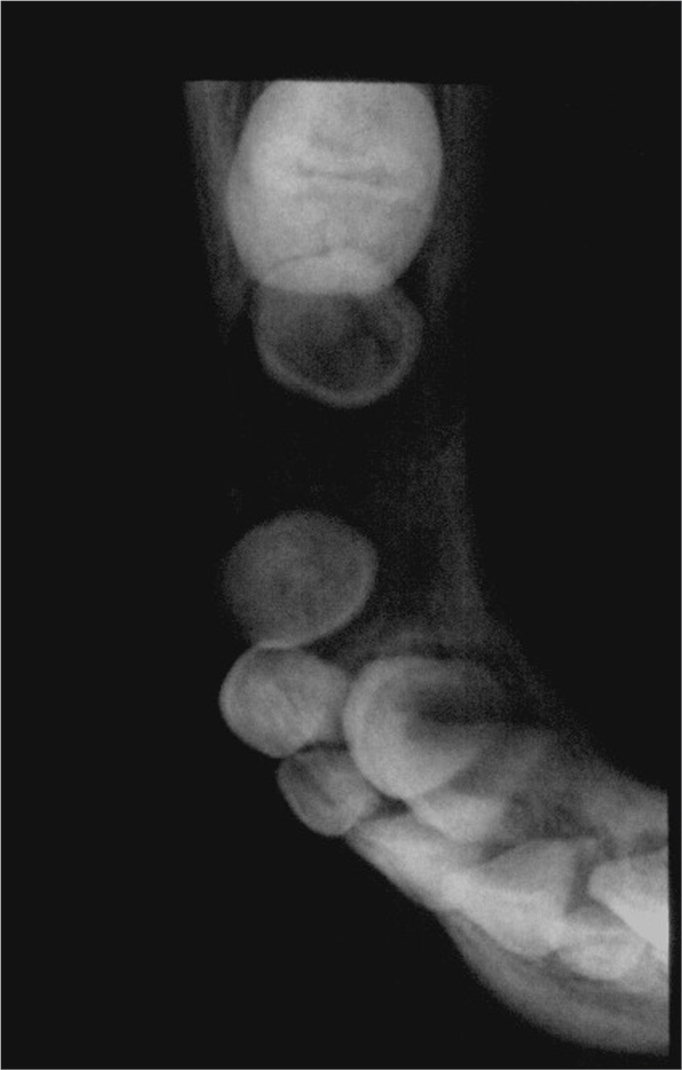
Burkitt's lymphoma - Occlusal radiograph - note dislocation of the premolar germs and rupture of the cortical layer.
